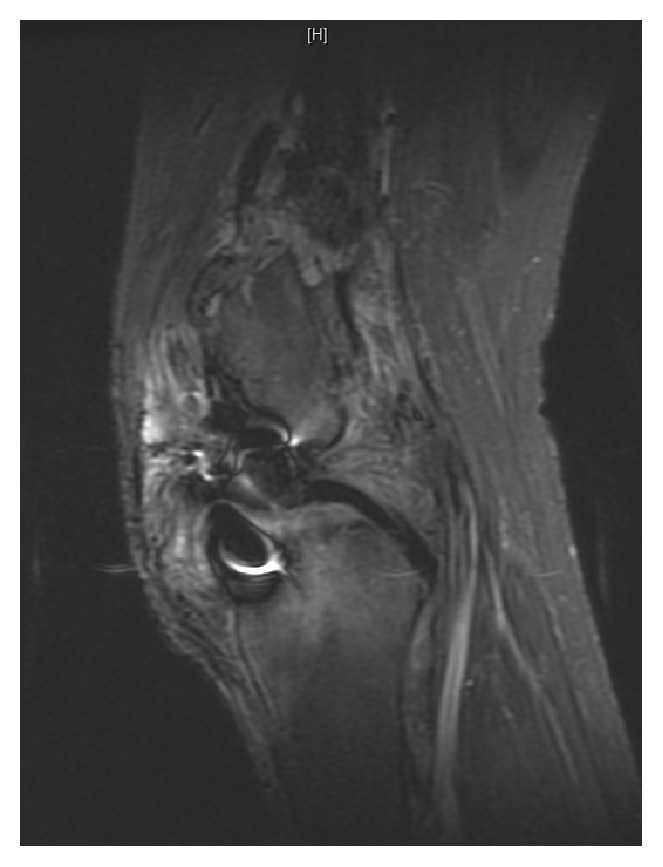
Sagittal STIR MRI of left knee with fracture nonunion of distal femur, marked synovitis, and bony changes consistent with osteomyelitis versus reactive changes.
